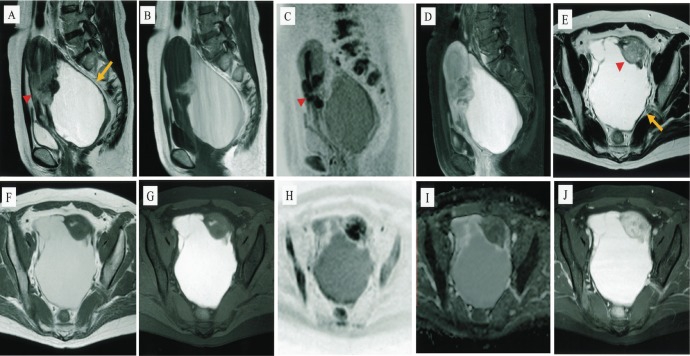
Magnetic resonance imaging findings. (A, E): T_2_-weighted images, (B, F) T_1_-weighted images, (G) fat suppression T_1_-weighted images, (C, H) diffusion-weighted images (DWIs), (I) apparent diffusion coefficient value map, (D, J) gadolinium-DTPA enhanced T_1_-weighted images. Solid mass in uterus (arrowhead) was hyperintense on T_2_WI, isointense on T_1_WI, heterogeneously enhanced on gadolinium-DTPA-enhanced T_1_WI, and hyperintense on DWI. Its apparent diffusion coefficient value was 0.9 × 10^−3^ mm^2^/s. The cystic portion (arrow) had an irregular wall and a septum protruding into the solid mass of the posterior wall. Its contents appeared to be old hemorrhagic matter with precipitated clot-like material. DTPA, diethylene triamine pentaacetic acid.
